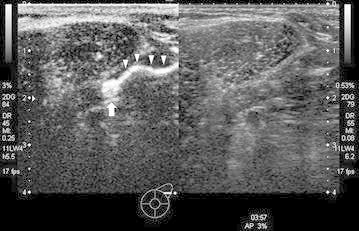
An Axillary lymph node detection using contrast-enhanced ultrasonography with Sonazoid injected subareolarly. A single route/single SLN pattern in a 64-year-old woman with 18 × 17-mm tumor in the left lower outer quadrant area, where only a single common lymph vessel from the subareolar area (*white arrow head*) drains into a single common SLN (*white arrow*)
